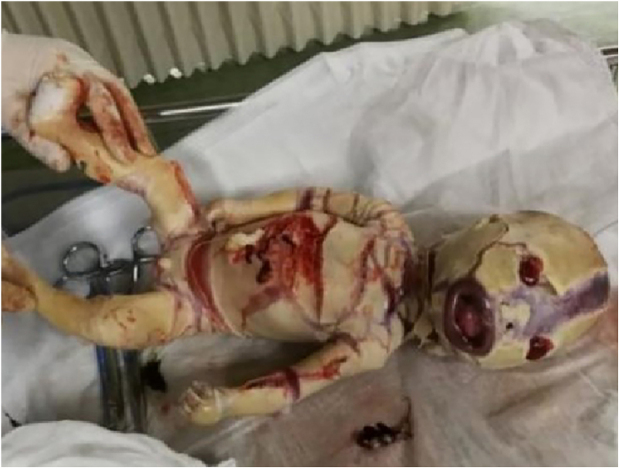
The appearance of abortus with Harlequin Ichthyosis was consistent with our prenatal ultrasound diagnosis
